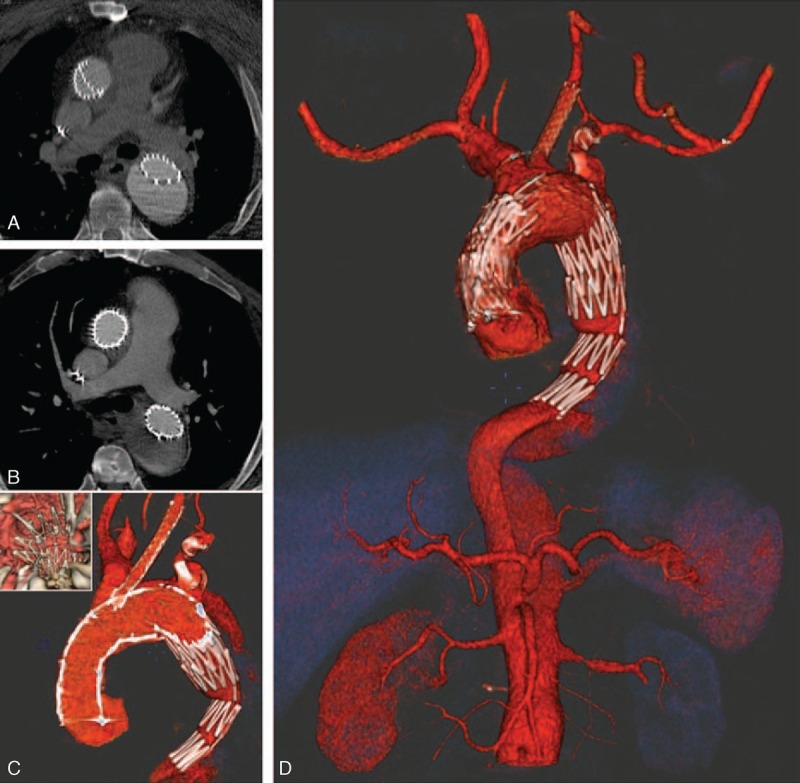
(A) Angio-CT documenting the evolution of the proximal infolding; (B) angio-CT documenting the resolution of the proximal infolding by the well-expanded Jotec stent; (C) final volume rendering of the arch; (D) final volume rendering of the aorta. CT = computed tomography.
